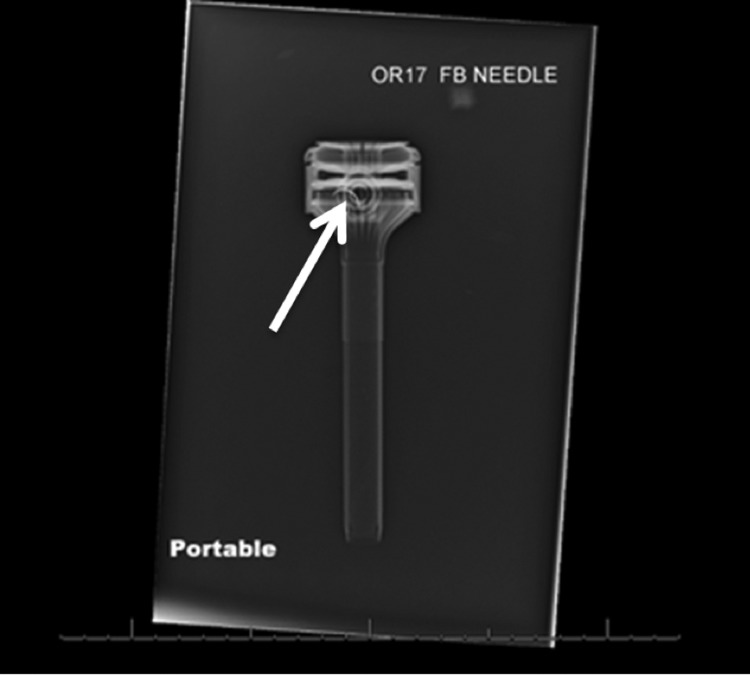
Portable radiograph showing needle in trocar head (*white arrow*).
